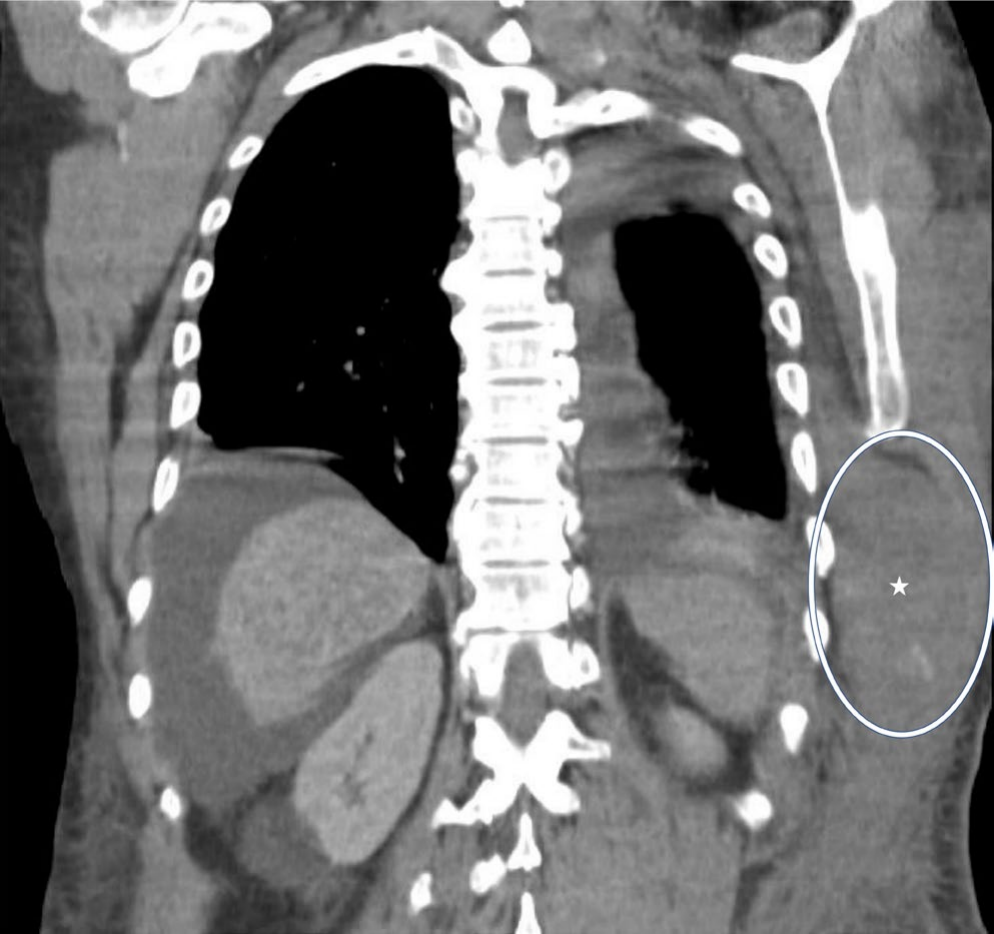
A computed tomography of the chest and abdomen in the coronal view showing a large intramuscular hematoma within the left lateral chest wall and extending to the upper abdomen, denoted by a star and a circle
